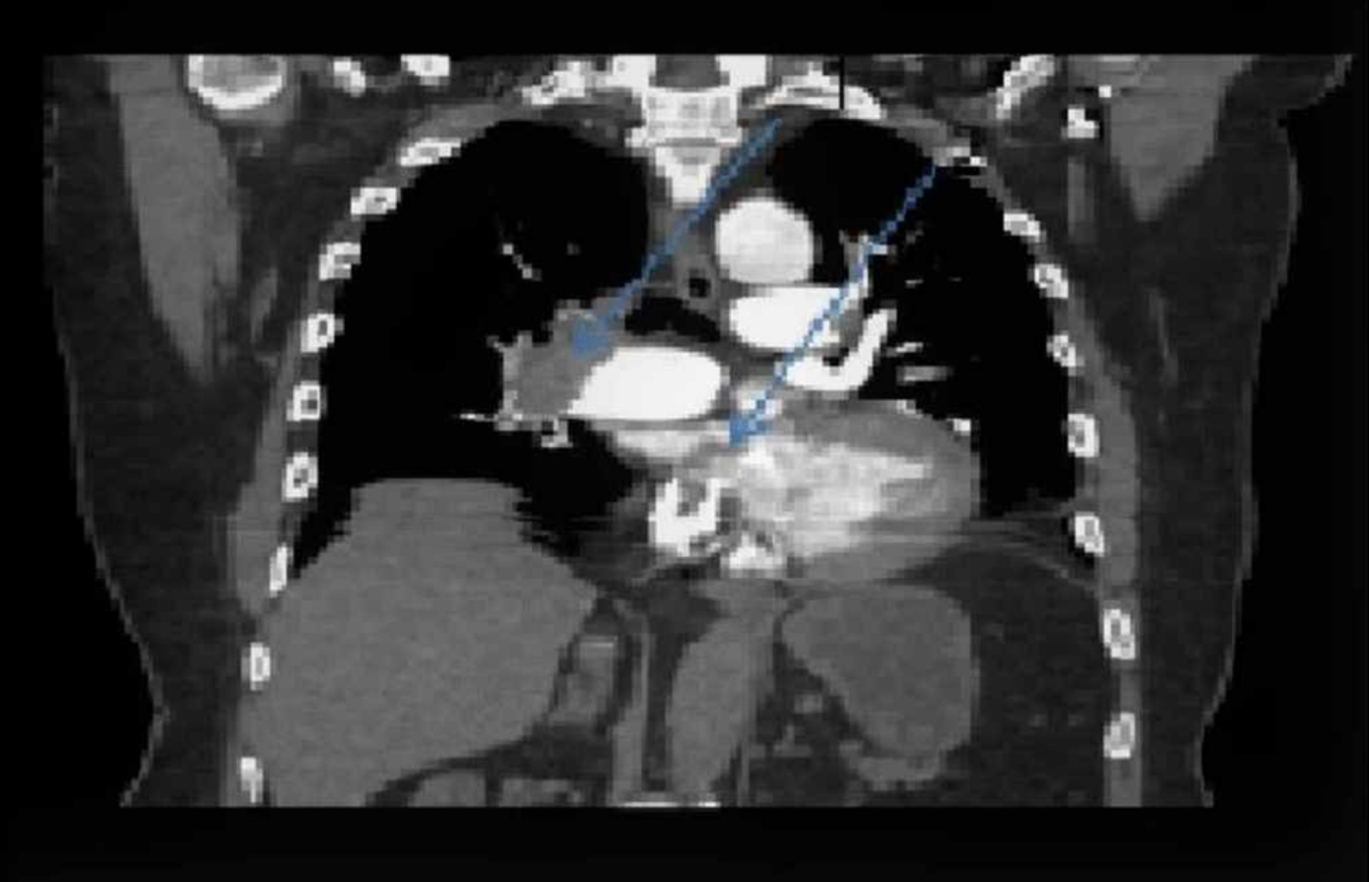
Computed tomography (CT) scan of the chest with intravenous (IV) contrast, coronal section. 1. Right main pulmonary artery occluded with thrombus. 2. A clot is clearly visible to be traversing from the right atrium to the left through the patent foramen ovale (PFO).
